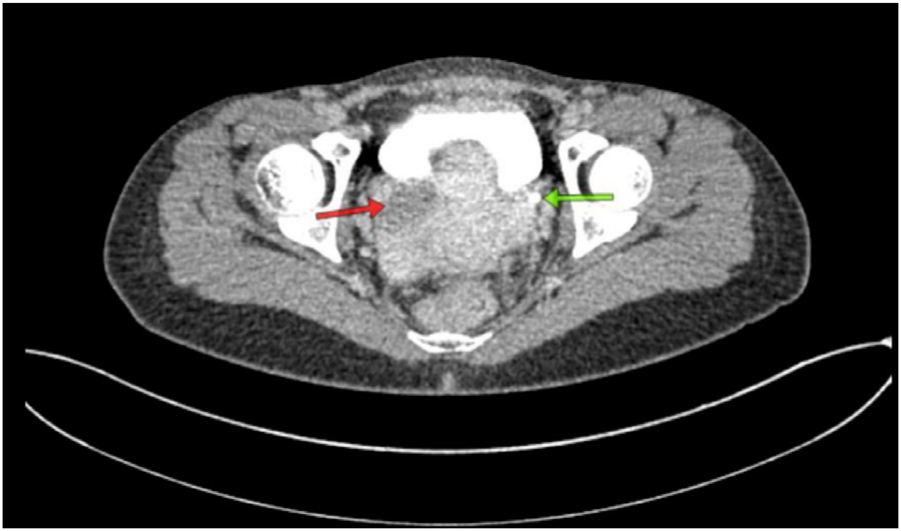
Contrast-enhanced abdominal CT scan: The contrast does not appear to fill the entire right ureter due to the size of the right ureter which is dilated (dilated/hydroureter) and tortuous, while the left ureter appears to have contrast passing through and not flowing into the urinary bladder.
